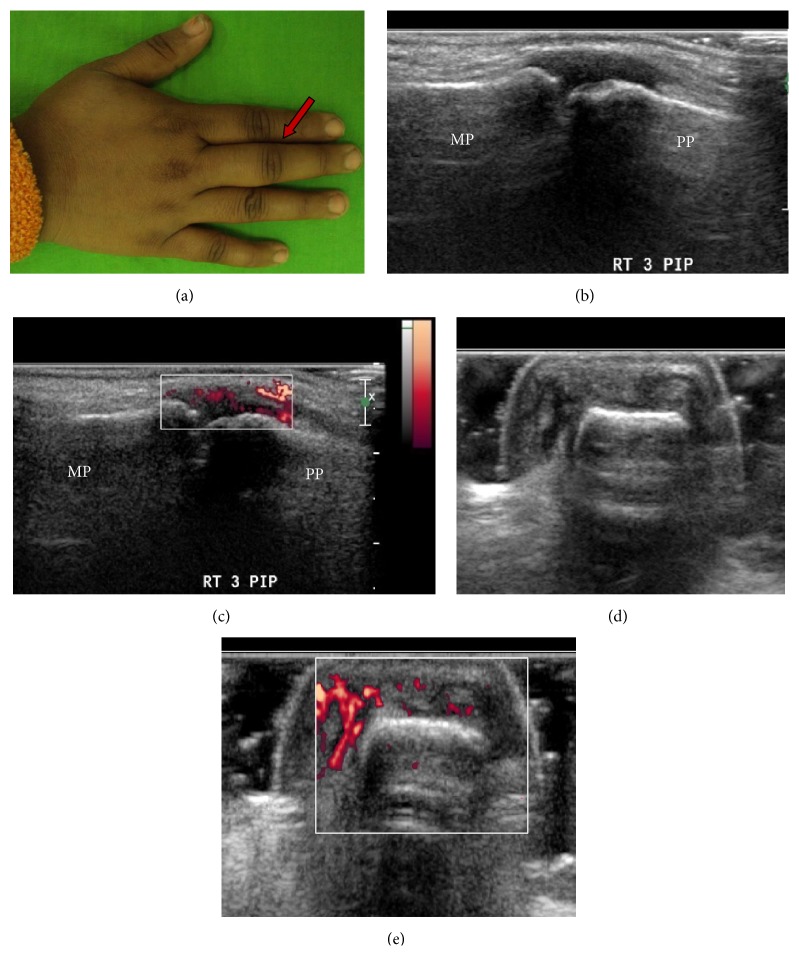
A 30-year-old female patient diagnosed as PsA presented with history of pain and swelling of right third PIP (arrow) (a). DAS28 score was 4.48 (suggestive of moderate disease activity). Ultrasound examination revealed Grade 2 synovial hypertrophy in right third PIP joint (b and d). PD evaluation showed increased signal (Grade 3) within the hypertrophied synovium in the joint (c and e). US scores derived were as follows: GSJC-11, GSJS-18, PDJC-3, and PDJS-7. Longitudinal view (b and c). Transverse view (d and e).
